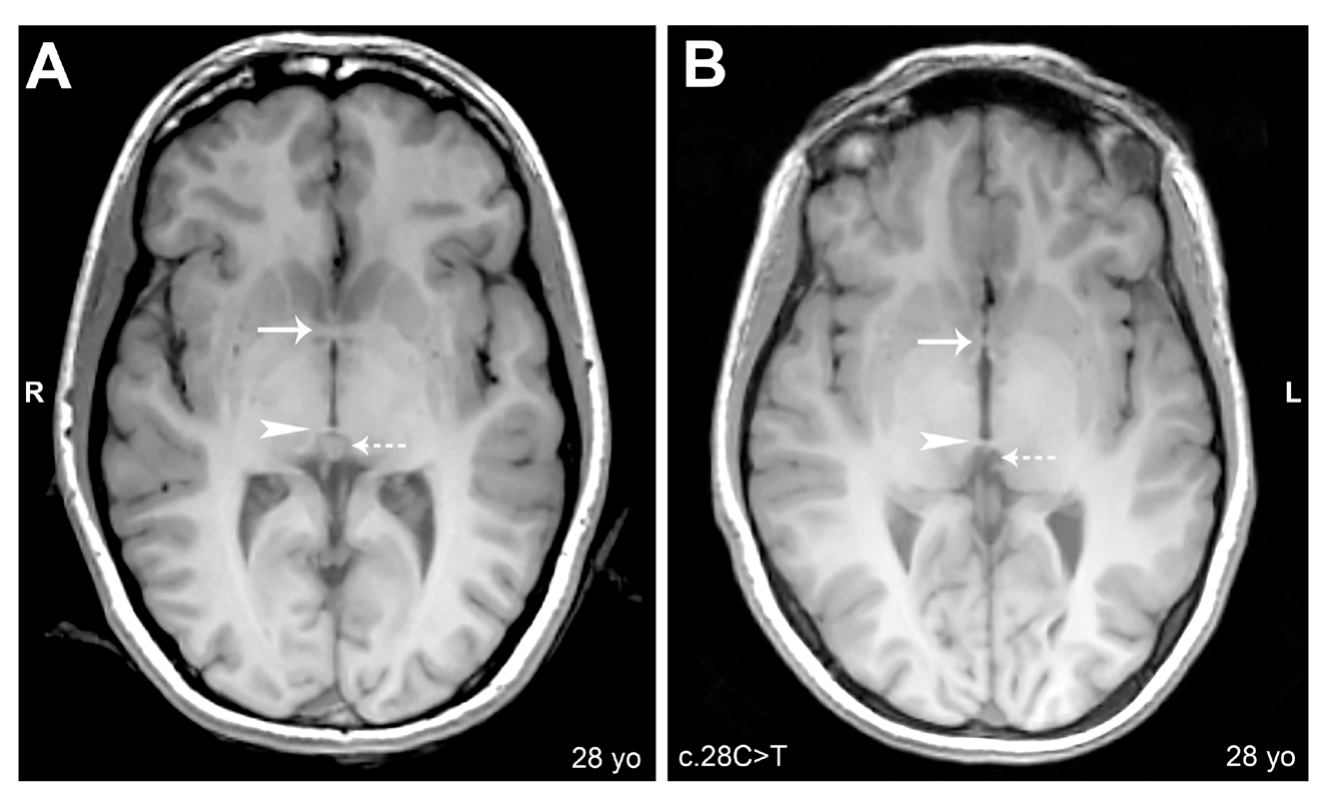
Anterior commissure, posterior commissure, and pineal gland: Axial cerebral T1-weighted magnetic resonance images, slice thickness 1.2mm. (
